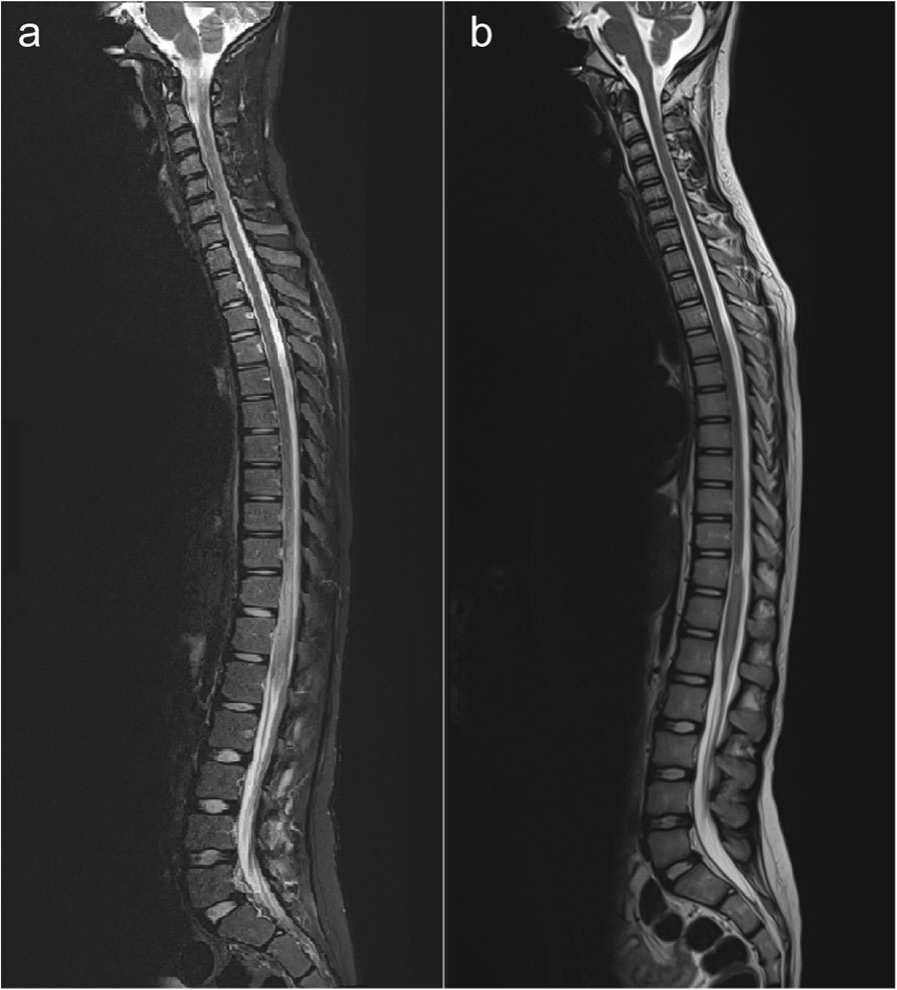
Demonstration of spinal cord schistosomiasis pre- and post-treatment. a Pre-praziquantel treatment. Sagittal T2-weighted magnetic resonance imaging revealed a circumferential and uniformly enlarged caudal spinal cord, including the thoracolumbar spine, in addition to a hyperintense signal intensity relative to the normal appearance of the cranial part of the spinal cord. b Post-praziquantel treatment. Sagittal T2-weighted magnetic resonance imaging revealed a significant regression of cord enlargement as well as high signal intensity in the caudal spinal cord
